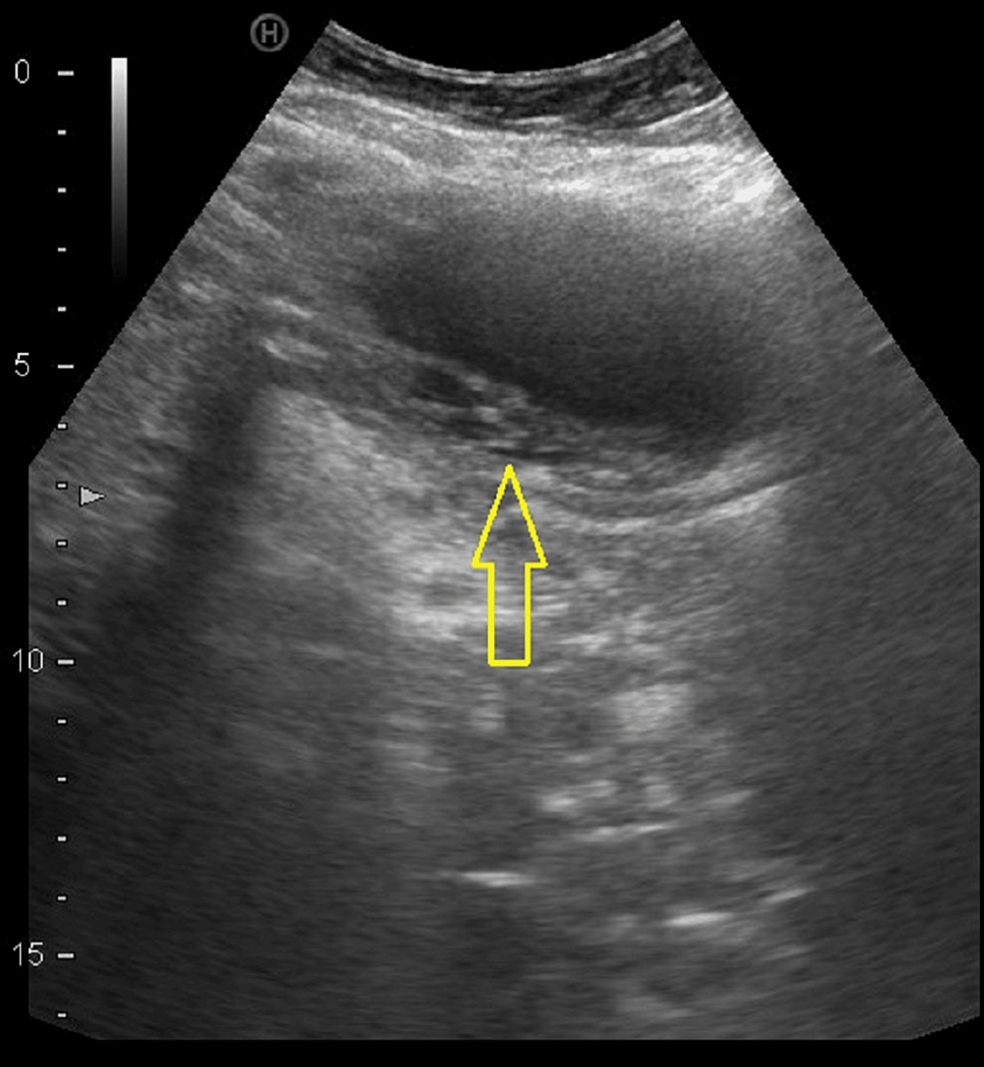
Ultrasonography findings that suggest acute cholecystitis (echogenic membranes inside gallbladder and wall thickening >3 mm).
